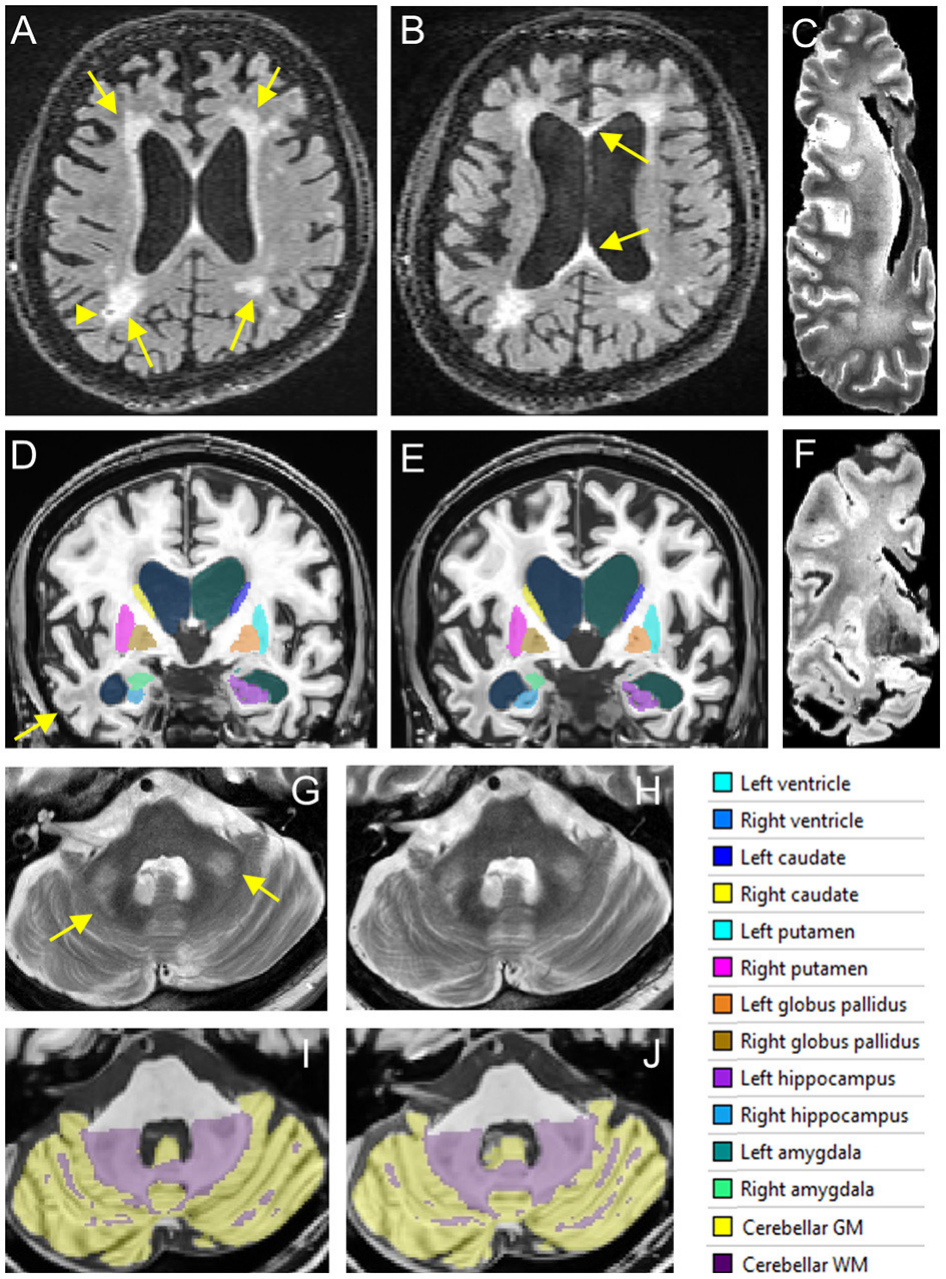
In vivo* and postmortem MRI showing white matter lesions, brain atrophy, and enlarged ventricles. (A–C) FLAIR scans acquired in 2016 (A) and 2017 (B) show periventricular WMHs (arrows in A) and a lacune (arrowhead) in right parietal white matter. The corpus callosum is thinned and has WMHs in both genu and splenium (arrows in B). WMHs become confluent in the frontal and parietal regions in the postmortem T2 scan of the right hemisphere (C). (D–F) Segmentation of the lateral ventricles and subcortical nuclei on T1 scans acquired in 2016 (D) and 2017 (E). White matter lesions in the right temporal lobe are observed on both *in vivo* MRI scans (arrow) as well as the postmortem T2^*^ scan (F). (G,H) The MCP sign (arrows) on T2 scans acquired in 2016 (G) and 2017 (H). (I,J) Segmentation of the cerebellum on T1scans acquired in 2016 (I) and 2017 (J).
